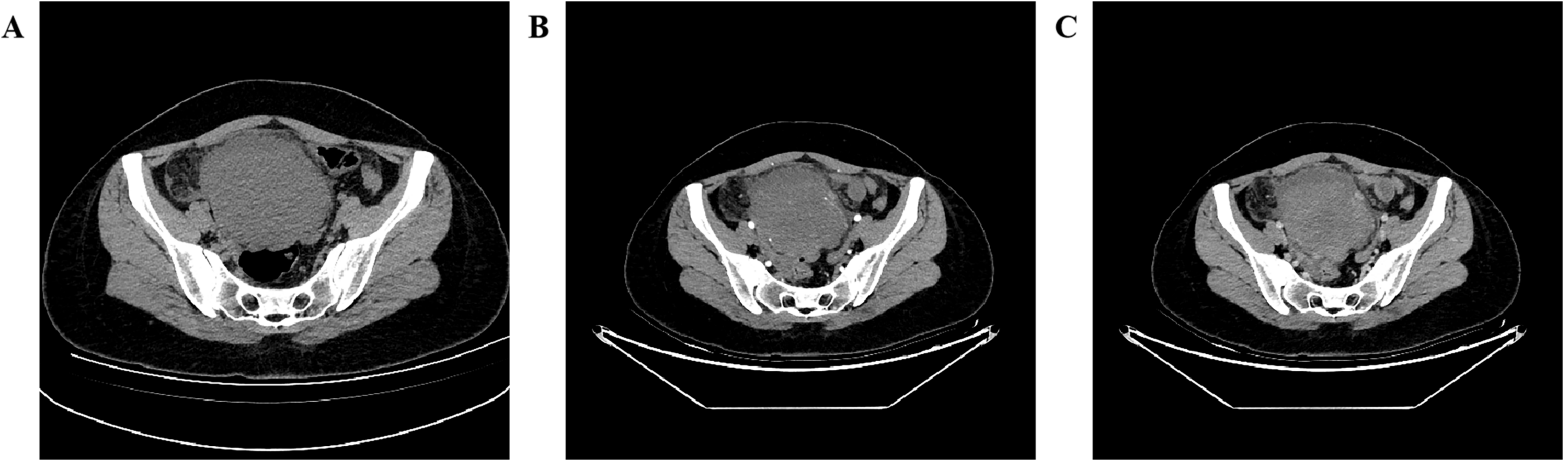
Pelvic CT axial view shows: (A plain scan, B enhanced arterial phase, C enhanced venous phase) A slightly hyperdense mass is seen in the pelvic cavity with ill-defined margins, blurred surrounding spaces, and heterogeneous enhancement, with more obvious enhancement in the upper part of the lesion.
